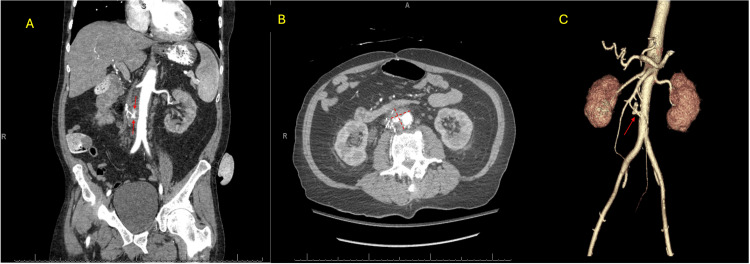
CT scan with 3D reconstruction demonstrating active extravasation A) Coronal view demonstrating active extravasation noted by the bright contrast (red arrows) B) Axial view that demonstrates the aortocaval hematoma (red arrows) that measures 3.2cm x 2cm. C) 3D reconstruction of IVC filter penetrating the aorta (red arrow).
